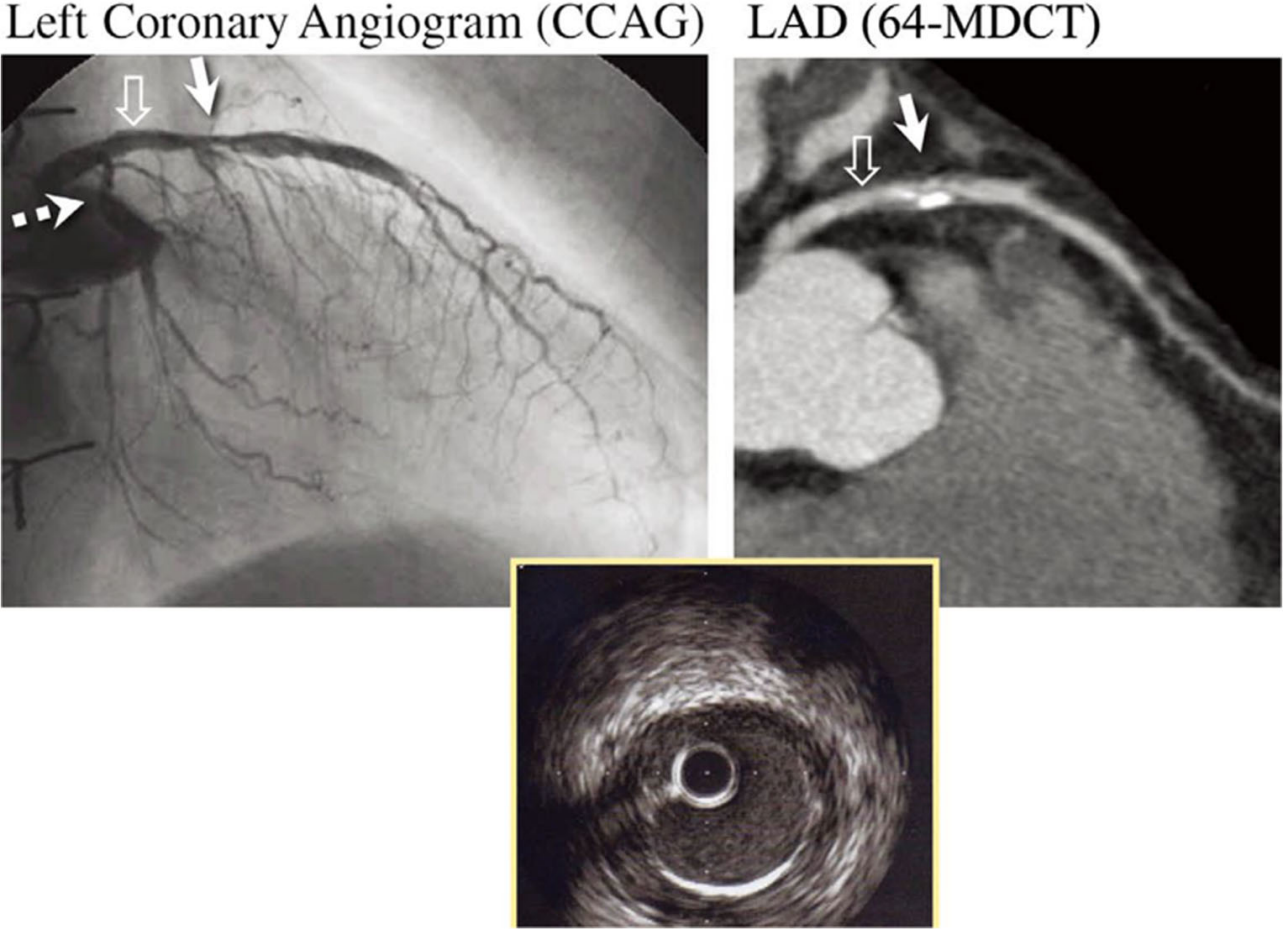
Coronary stenosis in the proximal–middle LAD (solid arrows) revealed by both invasive and CT coronary angiography in a heart transplant recipient. Intravascular ultrasounds performed simultaneously confirmed intimal hyperplasia in the proximal LAD (open arrows). Used with permission from Nunoda et al. Circ J. 2010 May;74 [5]:946–53
